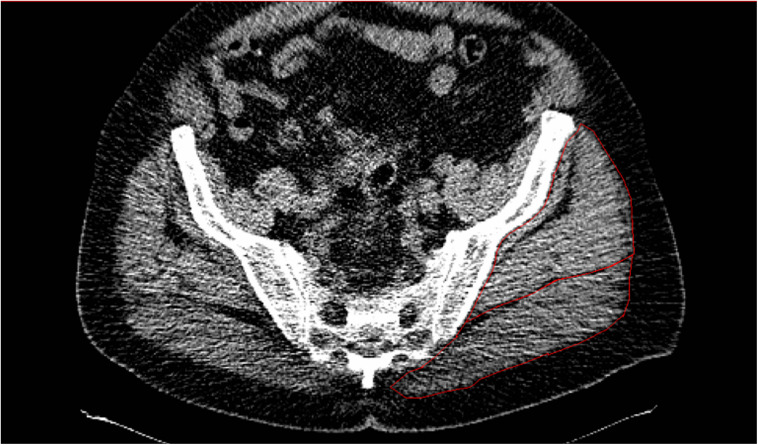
CT scans of one individual demonstrating low attenuation and high levels of IMAT in the gluteal muscles (outlined in red).
